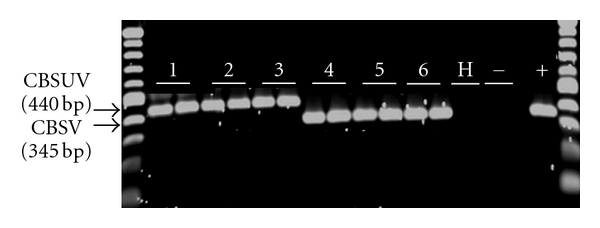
RT-PCR amplification of CBSUV and CBSV genomes using newly designed primers (CBSVF2 in combination with CBSVR7 and CBSVR8). 1 = CBSUV-[UG:Kab4-3:07], 2 = CBSUV-[TZ:Kib10-2:03], 3 = CBSUV-[KE:Mwa16-2:08], 4 = CBSV-[TZ:Zan6-2:08], 5 = CBSV-[MZ:Nam1-1:07], 6 = CBSV-[TZ:Nal3-1:07], H = RNA extraction from a CBSD-free plant, − = negative water control, and + = a known CBSV RNA control from previous sample preparations. The size ladder at each border of the gel is the 100 bp molecular weight markers (New England Biolabs, UK).
